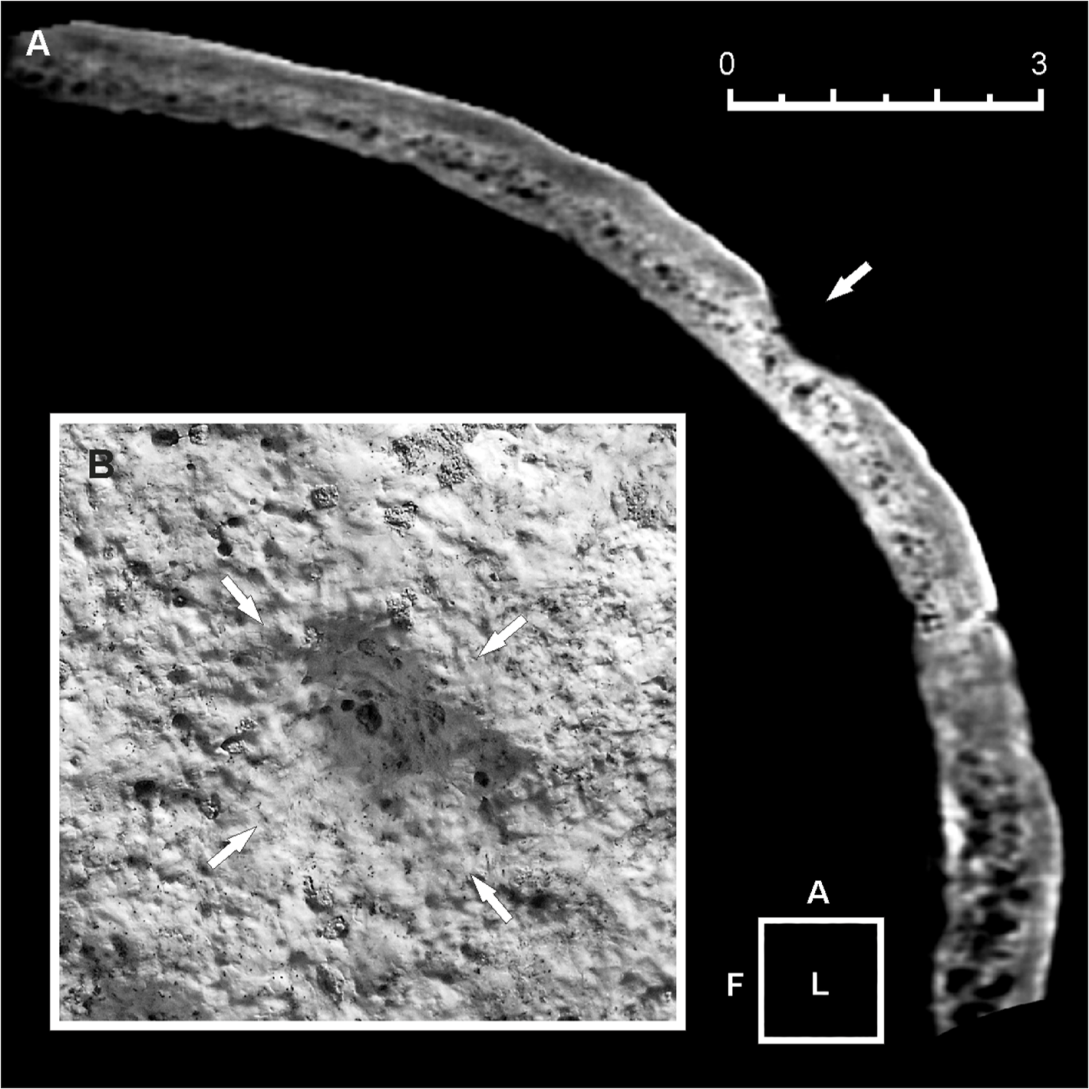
Three-dimensional (3D) computed tomography (CT) scan of the minor skull trephination. (A, B) Sagittal plane CT scan (A). Only the external cortical layer of the bone is affected (arrow). Detail of the cone-shaped lesion, identified as an incomplete trephination with a possible ritual purpose (B). Bone remodeling, hypervascularity and pitting of the ectocranial surface suggest an advanced phase of the bone healing process. Scale bar measures 3 cm.
